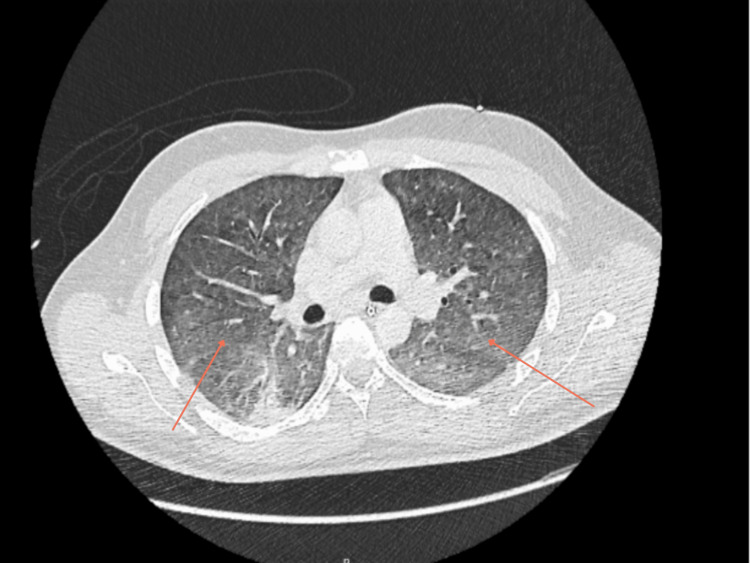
CT Thorax Abdomen Pelvis on day six Shows interval improvement in pulmonary opacities, however extensive bilateral diffuse ground glass component
